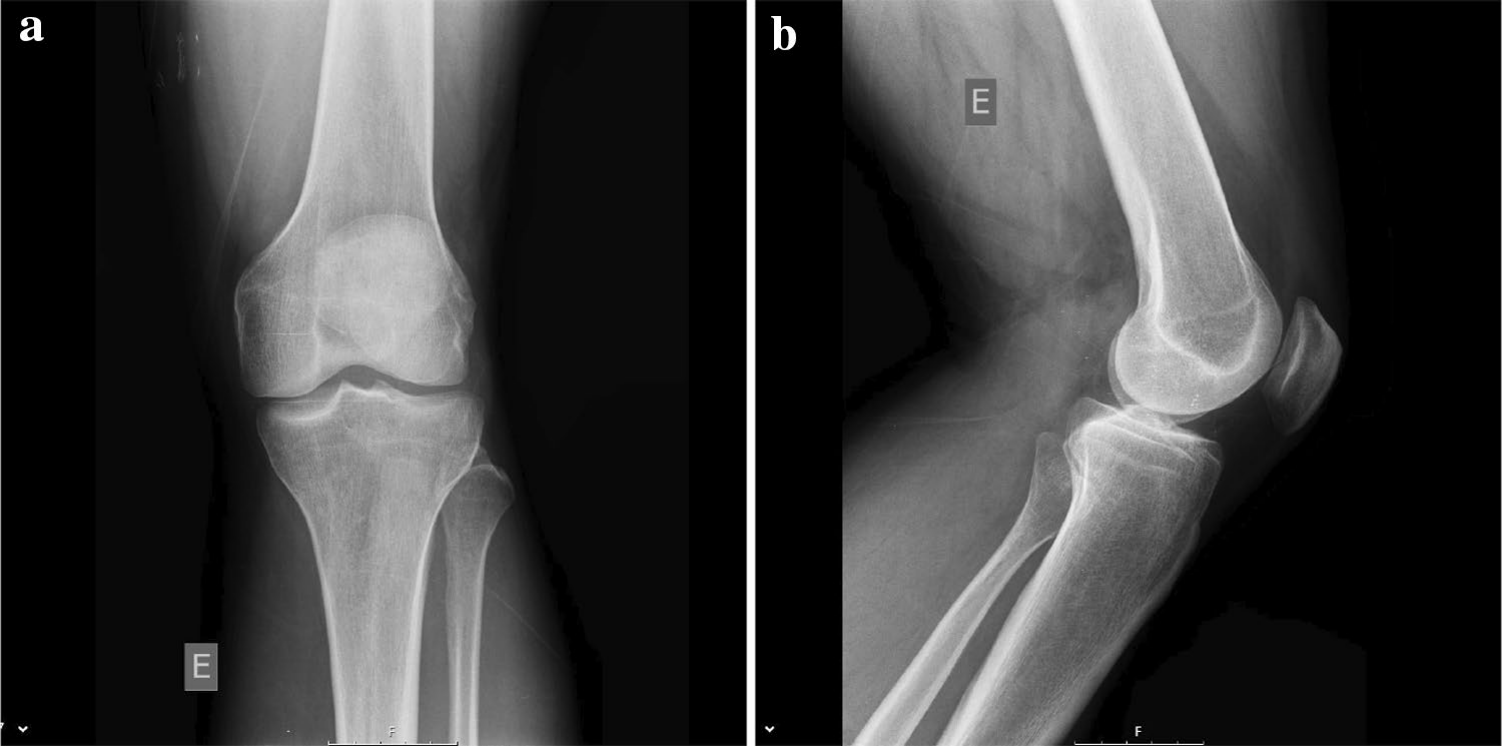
Left knee X-rays, where “E” is an indicator for “Left”: a normal anteroposterior view, and b lateral view
